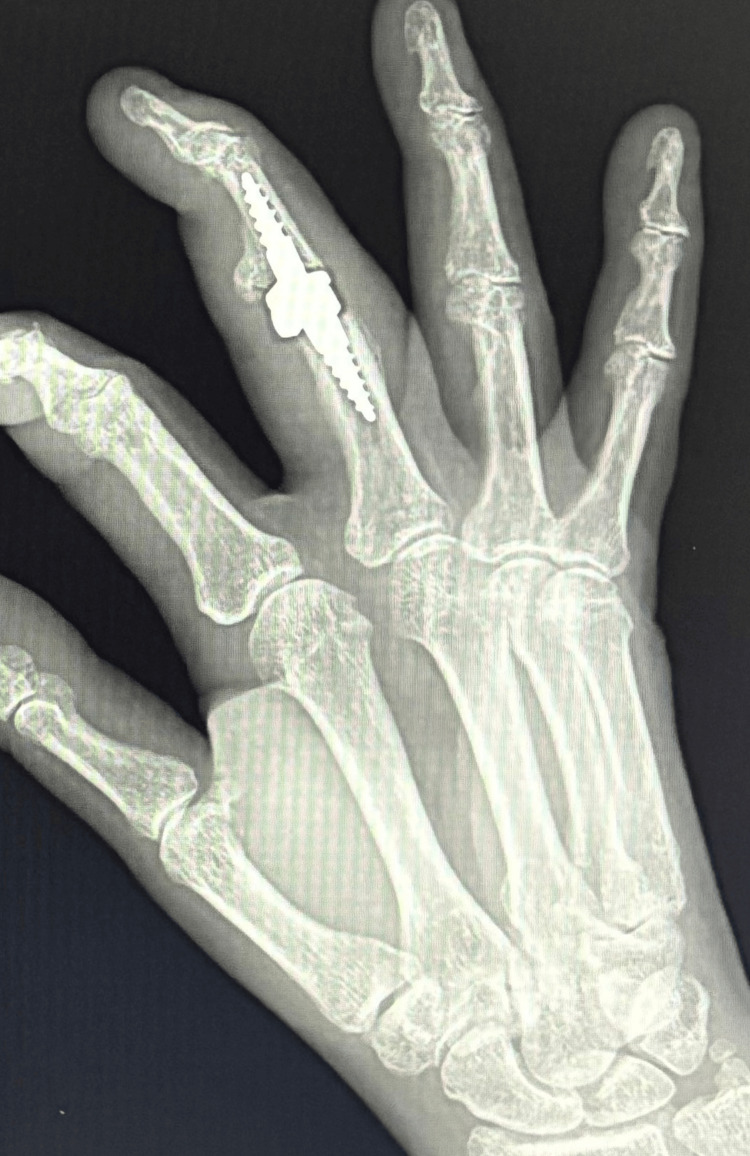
Control X-ray in Robert's projection six month after surgery
